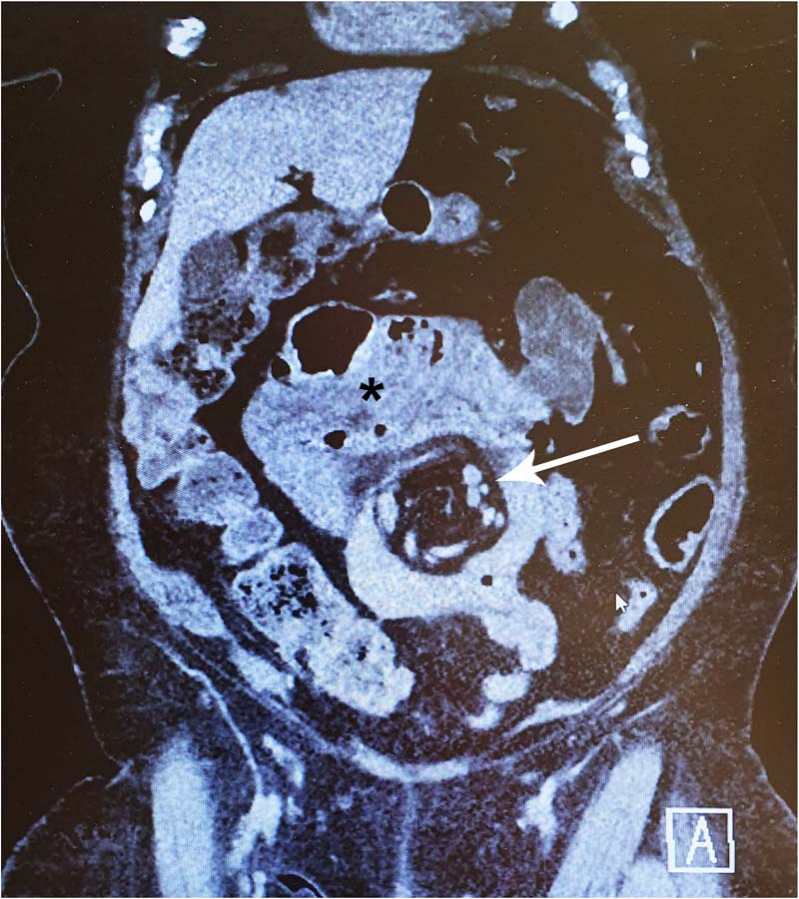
A 77-year-old woman with a history of jejunoileal bypass 31 years ago presented with intermittent colicky upper abdominal pain for 1–2 years. A rather painful episode led to hospital admission and CT scan, which showed a mesenteric swirl sign (arrow). Symptoms quickly subsided. Although she remained symptomless after that particular episode, an exploratory laparoscopy converted to laparotomy was performed 5 months later. This revealed a large mesenterial defect under the jejunoileal anastomosis. 390 cm of bypassed small bowel was found to be atrophied and packed with adhesions. This finding may be visible on CT scan (*).
